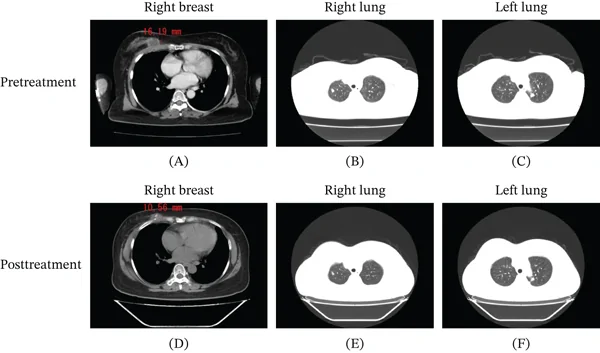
(A) CT image of the right breast tumor before treatment with docetaxel liposomes and SDT using verteporfin liposomes. (B, C) CT images of the right and left lungs, respectively, showing bilateral pulmonary metastases. (D−F) CT images of the right breast and bilateral lung fields obtained after treatment, demonstrating regression of the primary breast tumor and pulmonary metastatic legions.
